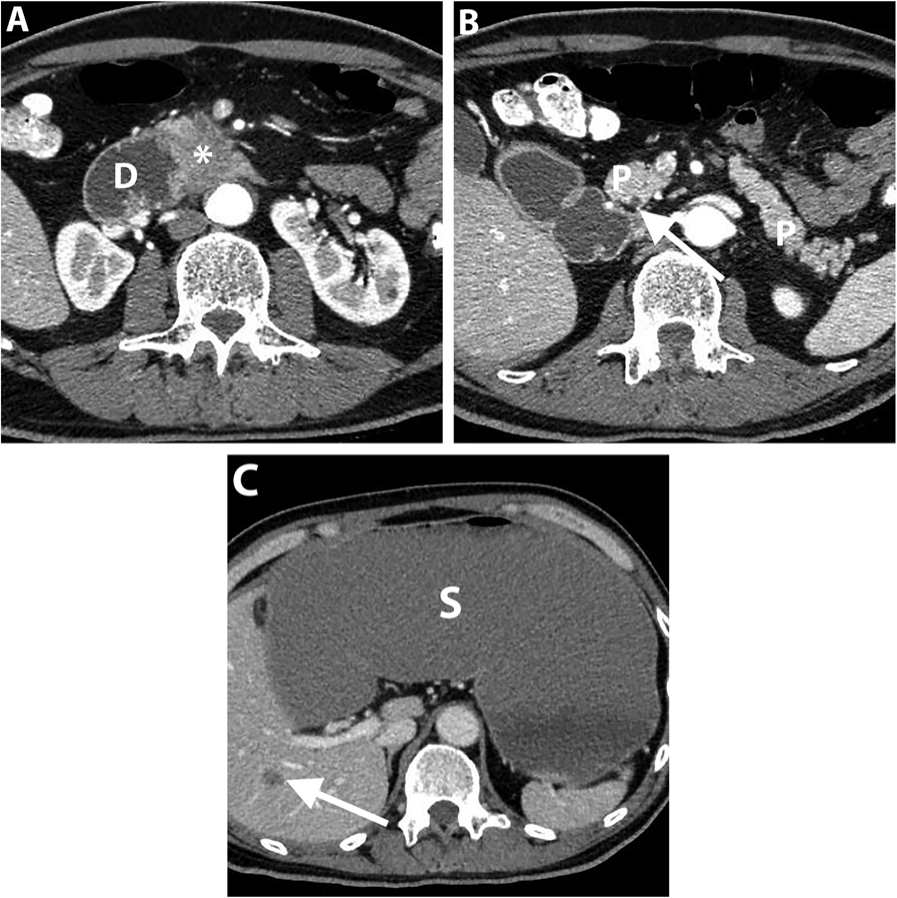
A 63-year-old man with uncinate process PDAC causing a duodenal obstruction. Axial pancreatic phase CT images (a and b) show a hypoattenuating mass (asterisk) arising from the uncinate process, infiltrating the third part of the duodenum (D) and abutting the anterior wall of the aorta. CBD (arrow) is normal in caliber. The pancreas (P) has no ductal dilatation or atrophy. Axial portal venous phase CT image (c) obtained 1 month later shows a marked distension of the stomach (S) due to duodenal obstruction and there is a new hypoattenuating metastatic liver lesion (arrow)
